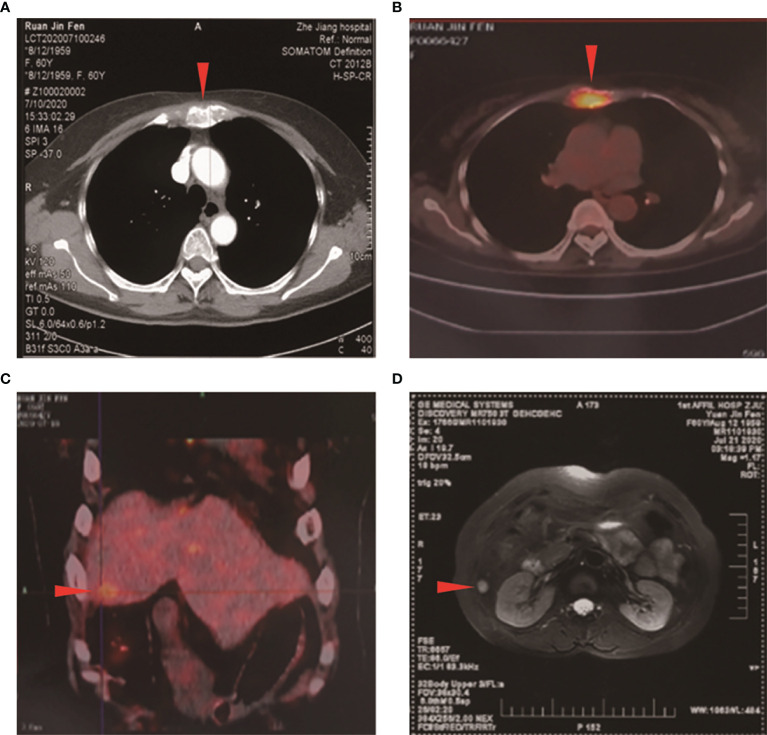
Initial computed tomography (CT), positron emission tomography/computed tomography, and magnetic resonance imaging (MRI) scans. (A) CT scan showed a space-occupying lesion in the mesosternum. PET-CT demonstrated high fluorodeoxyglucose (FDG) uptake in the (B) mesosternum and in the (C) right medial lobe of the liver (arrowhead). (D) MRI showed focal signal abnormalities in hepatic segment 8 (arrowhead).
